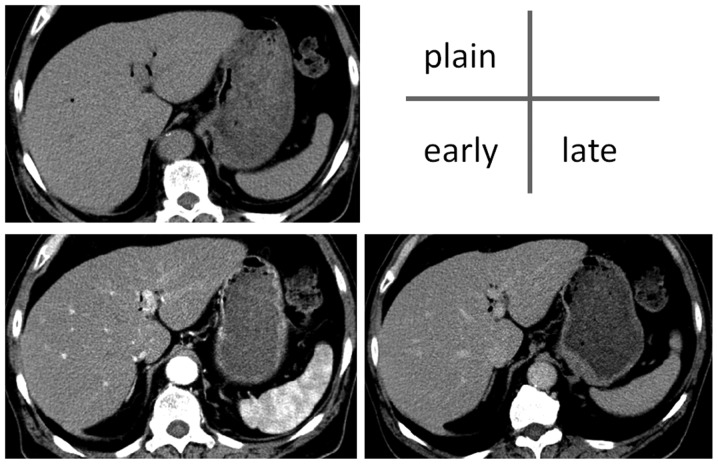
Abdominal computed tomography (CT) images on hospital day 7 showing no hepatomegaly, splenomegaly, ascites or distention of the intrahepatic or common bile ducts.
